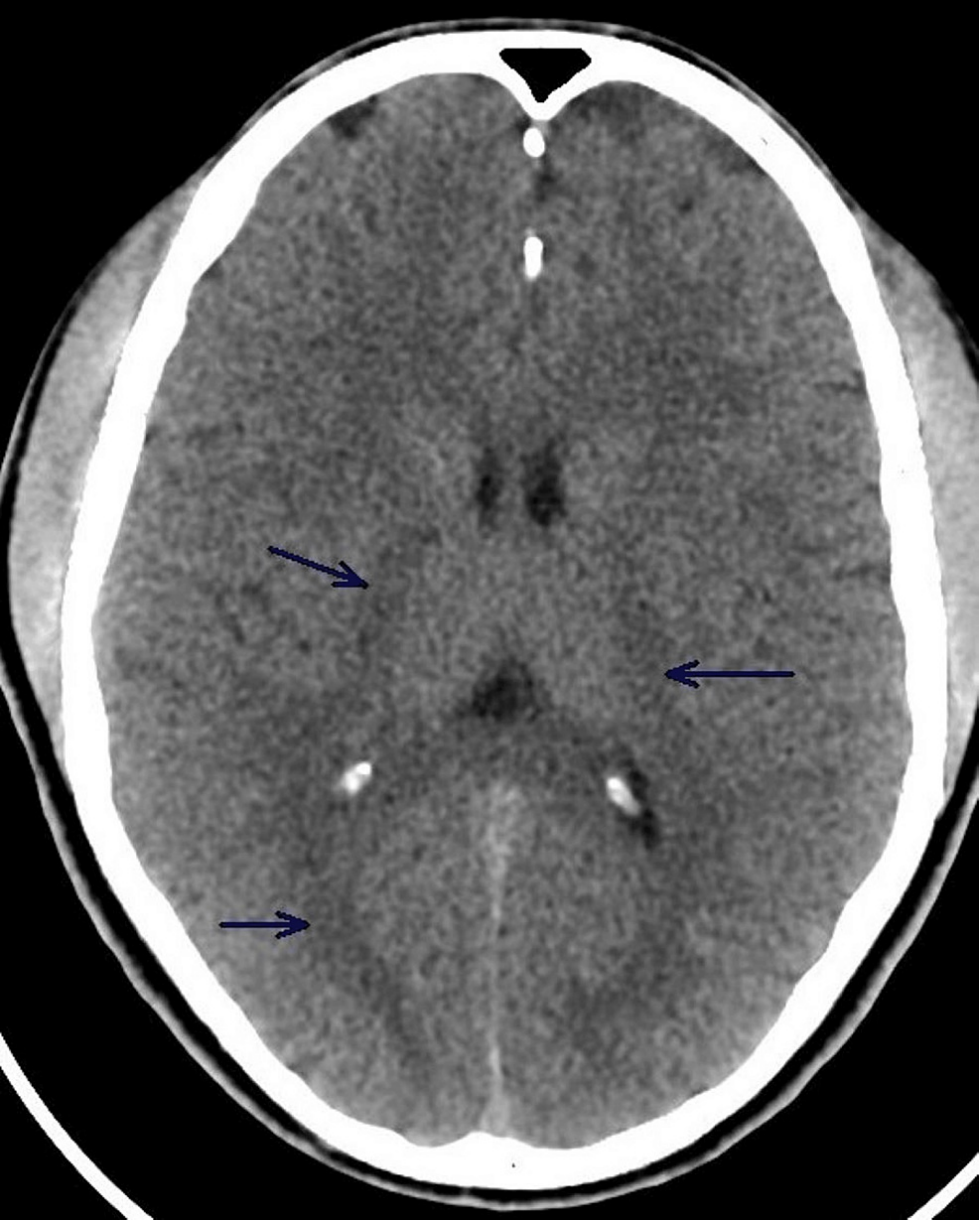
CT of the brain showing no acute abnormalities
